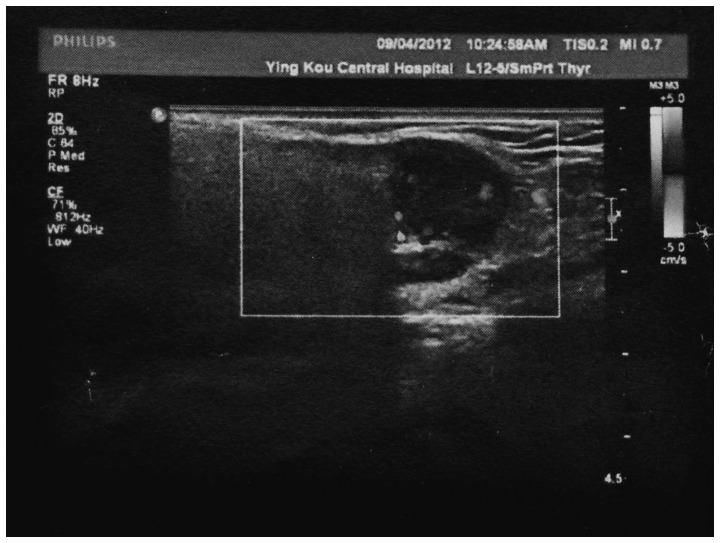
Ultrasonography of the parotid gland.
